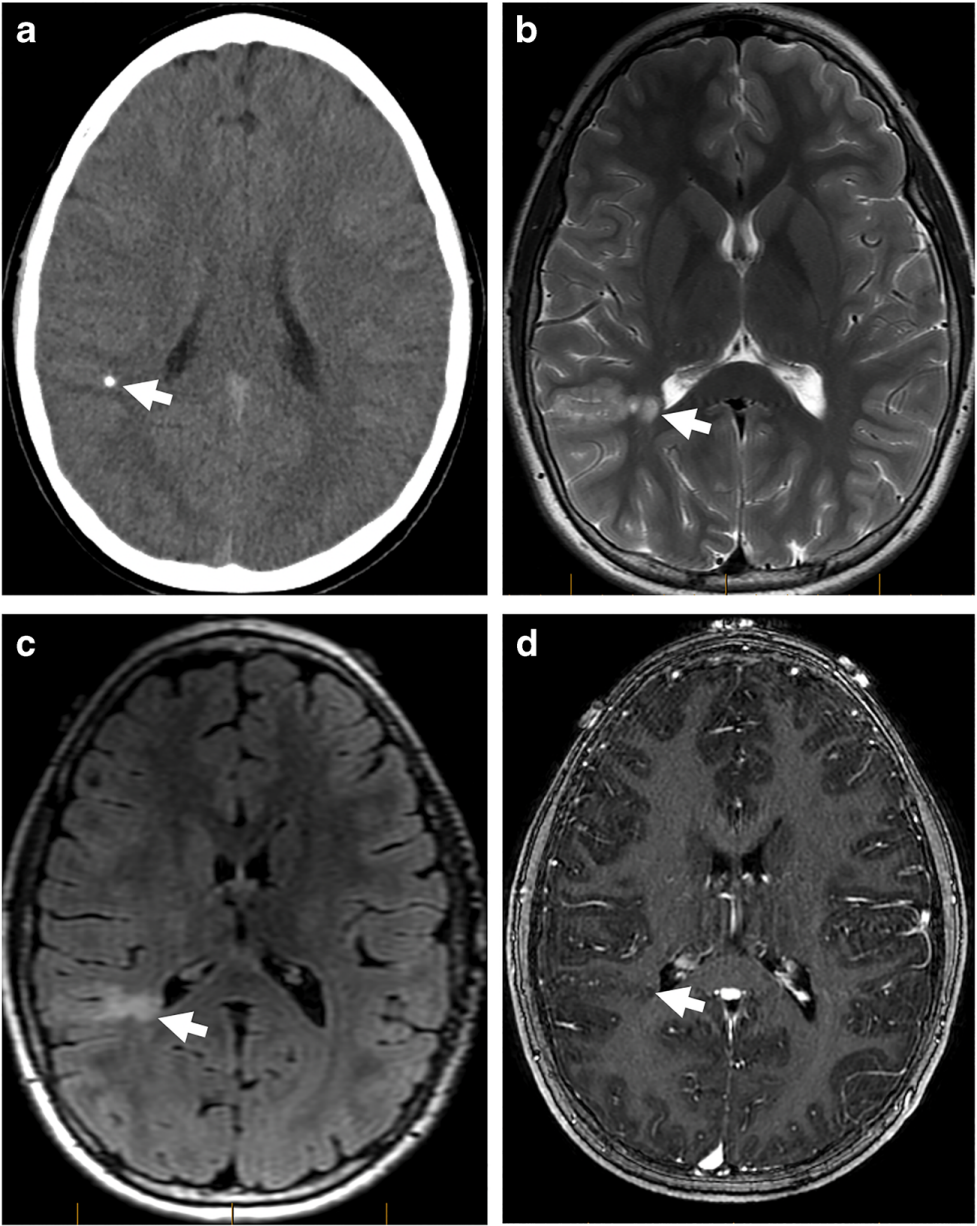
Polymorphous low-grade neuroepithelial tumor of the young. Macroscopic calcification (arrow) is characteristic on CT images (a). The tumor is hyperintense on T2 (b) and FLAIR (c), with no enhancement on the postcontrast T1-weighted image (d)
